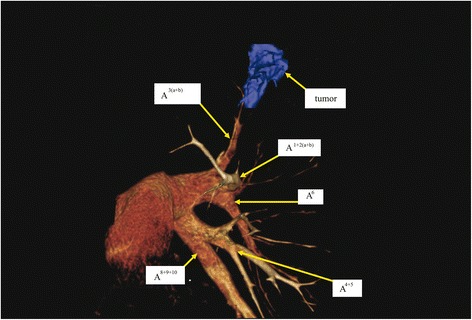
A preoperative 3D-CT angiographic image shows the left main pulmonary artery and the left mediastinal lingular and basal pulmonary arteries. The left A^4+5^ and A^8+9+10^ formed a common trunk
